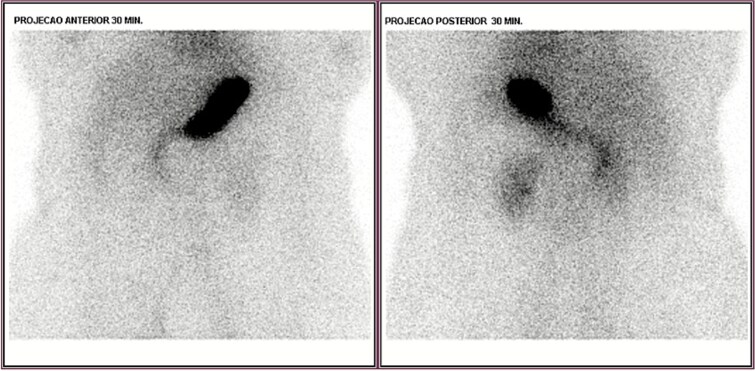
Negative scintigraphy for Meckel’s diverticulum.
